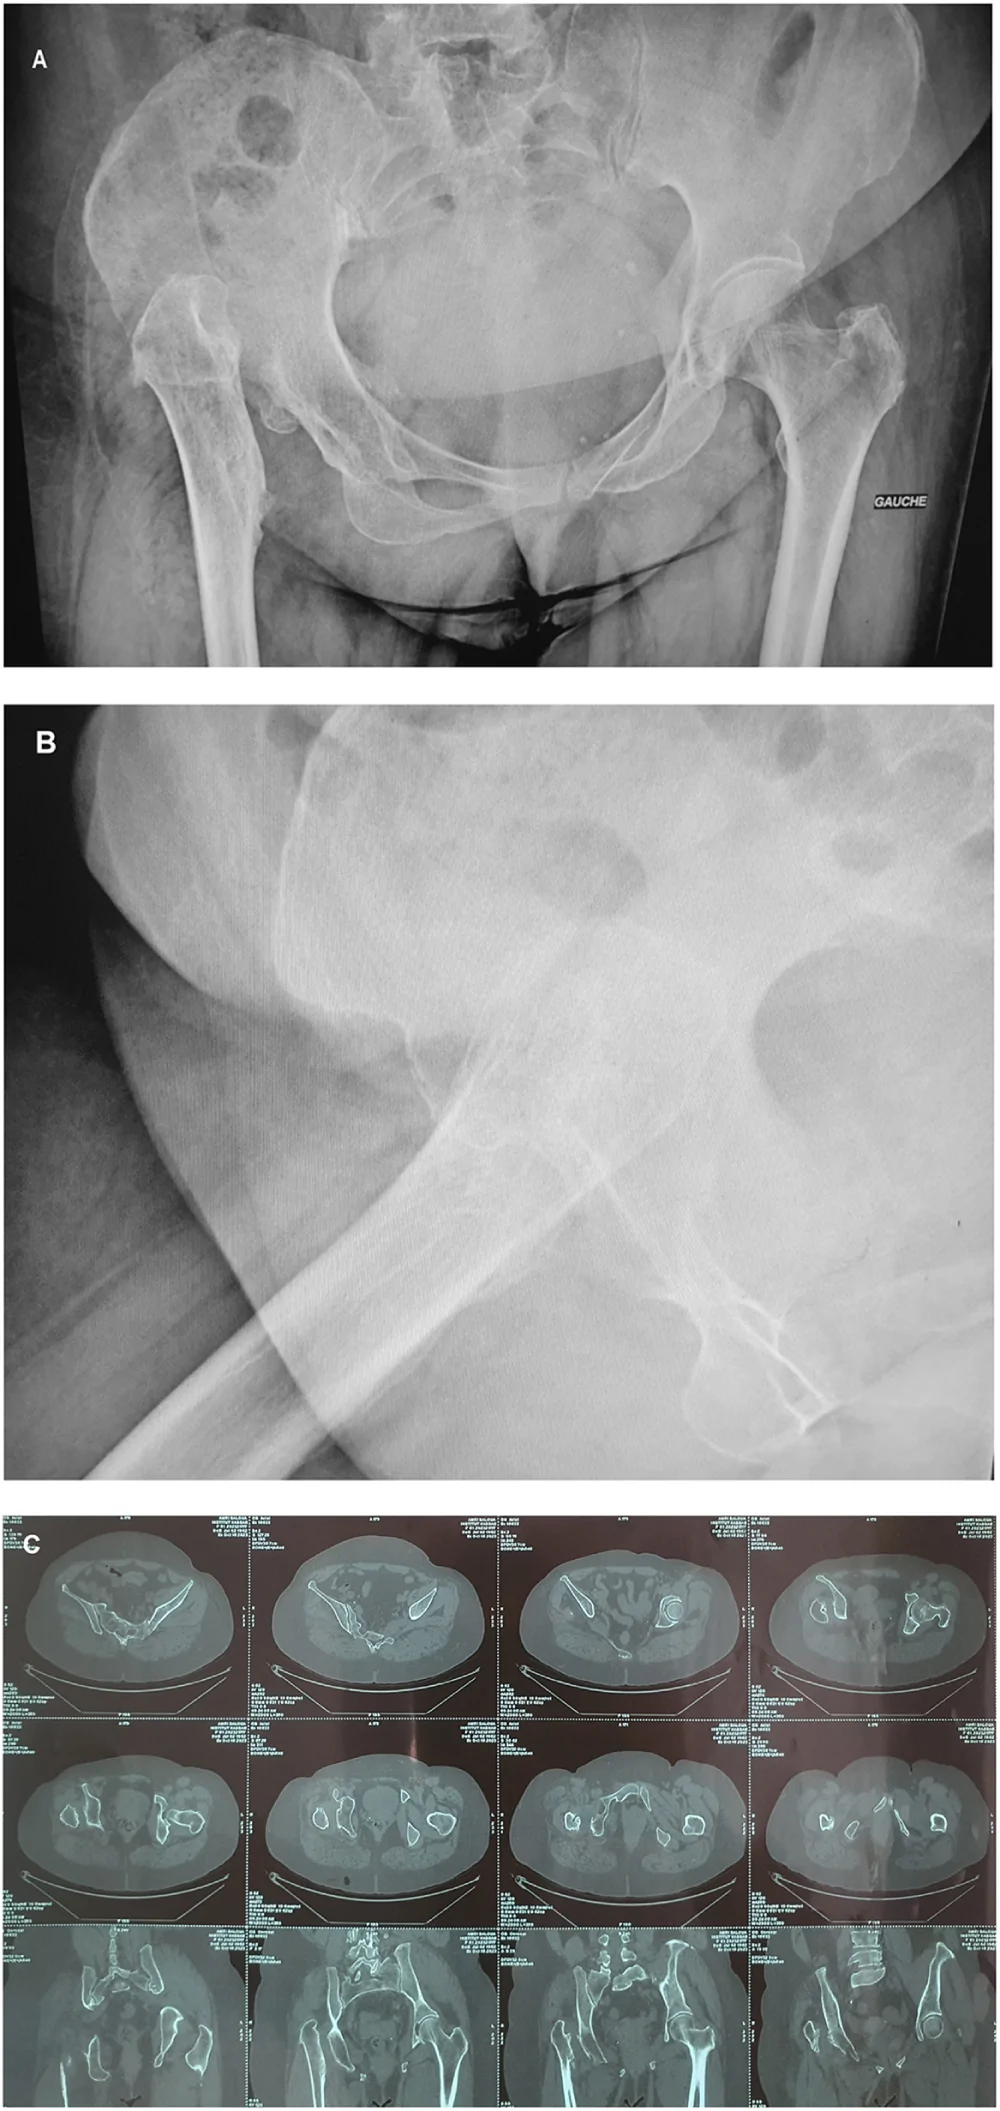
Preoperative anteroposterior (A) and lateral (B) radiographs of the pelvis showing a Crowe IV anterior hip dislocation. CT scan (C) showing complete resorption of the femoral head and neck and a dysplastic acetabulum.
